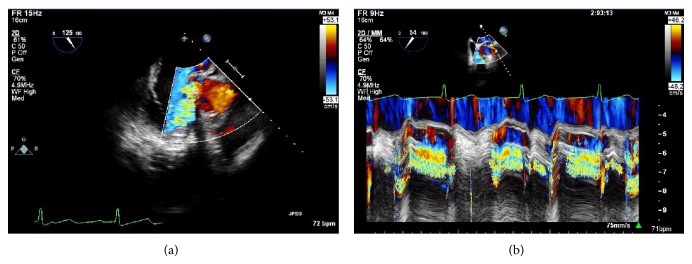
A transesophageal echocardiogram showing a bileaflet mechanical aortic valve with perivalvular abscess and valvular vegetation as well as severe eccentric paravalvular aortic regurgitation.
